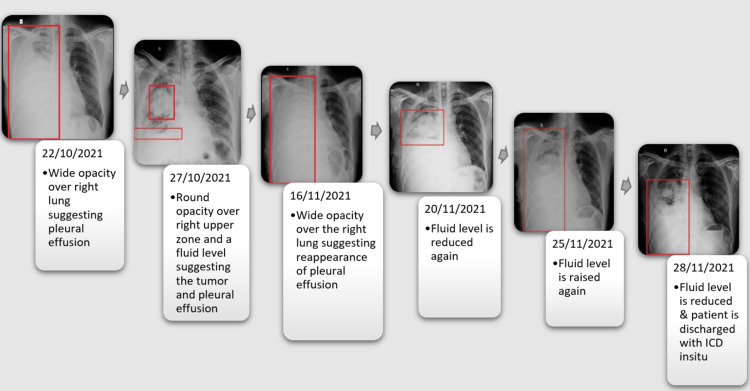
Chest X-rays of subsequent days showing the tumor along with recurrent pleural effusion ICD: Intercostal drainage
